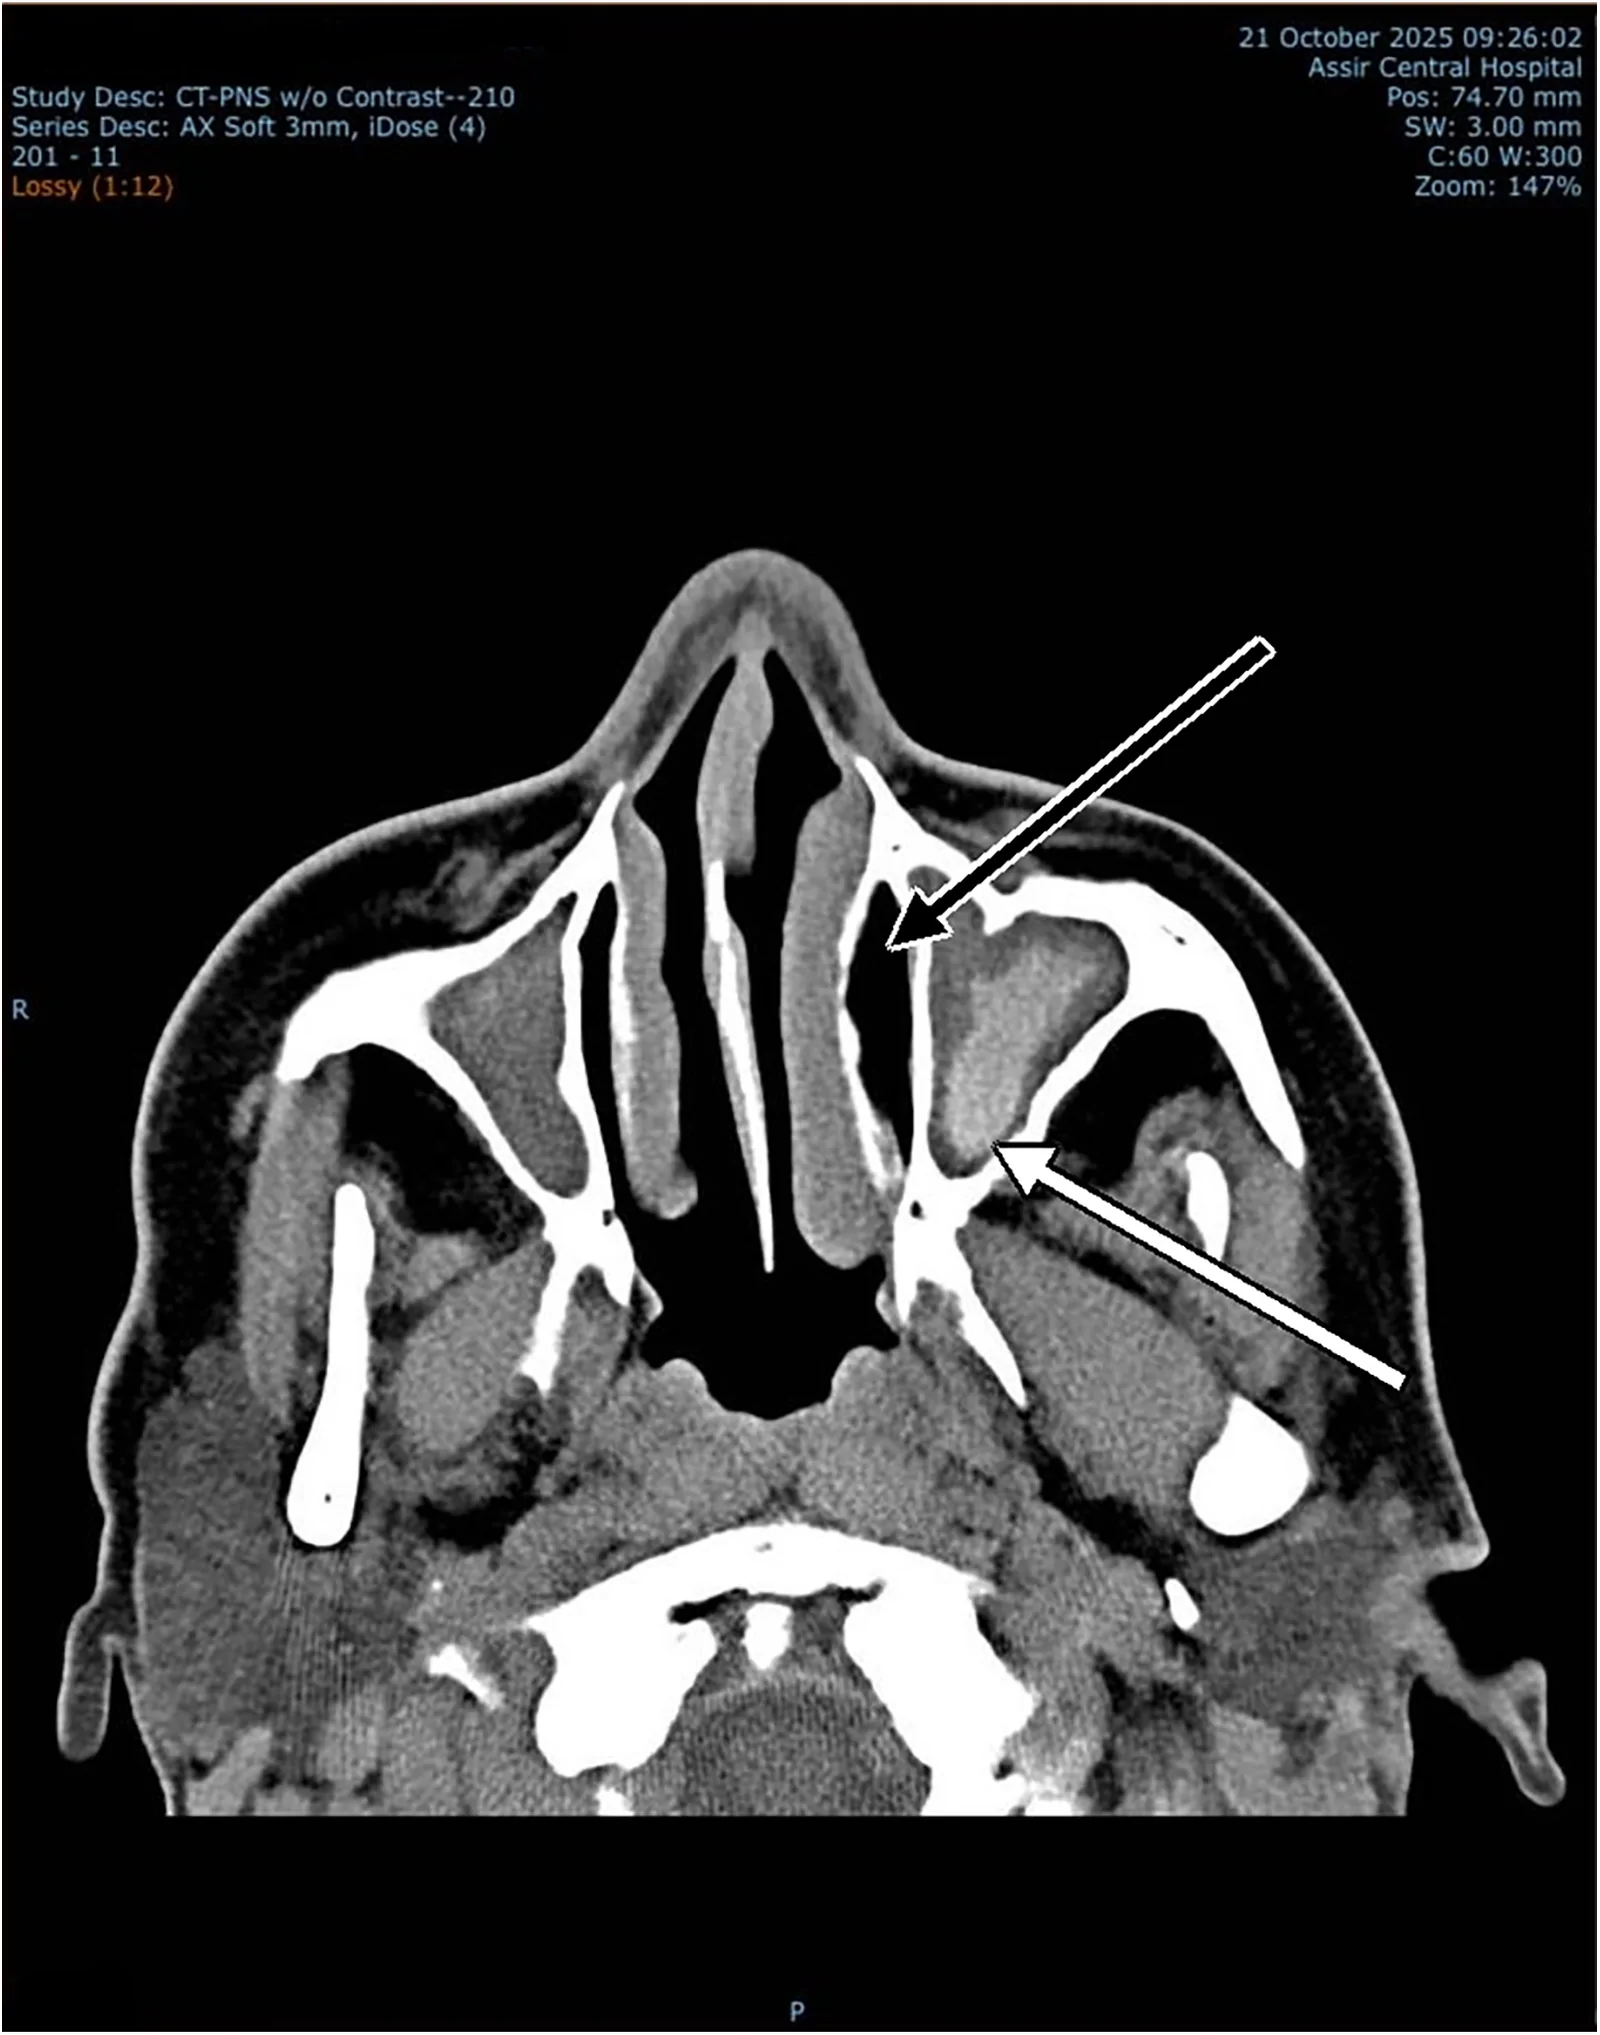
Axial CT of the paranasal sinuses (soft-tissue window), 22 October 2025, during anti-TSLP (tezepelumab) therapy, showing persistent sinonasal mucosal disease at the level of the maxillary sinuses and nasal cavity. Arrows indicate hyperdense material within the left maxillary sinus (white arrow) and remodeling of the left ostiomeatal complex (black arrow). Patient identifiers redacted.
